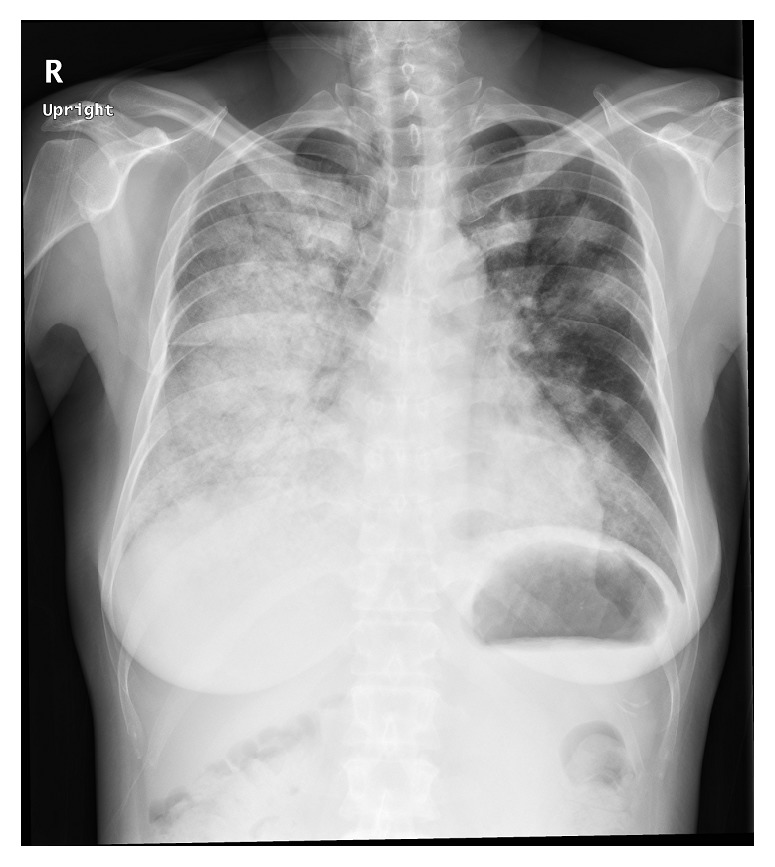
Chest radiograph upon the start of high-dose corticosteroid therapy. Infiltrative shadows are clearly observed in both lung fields.
